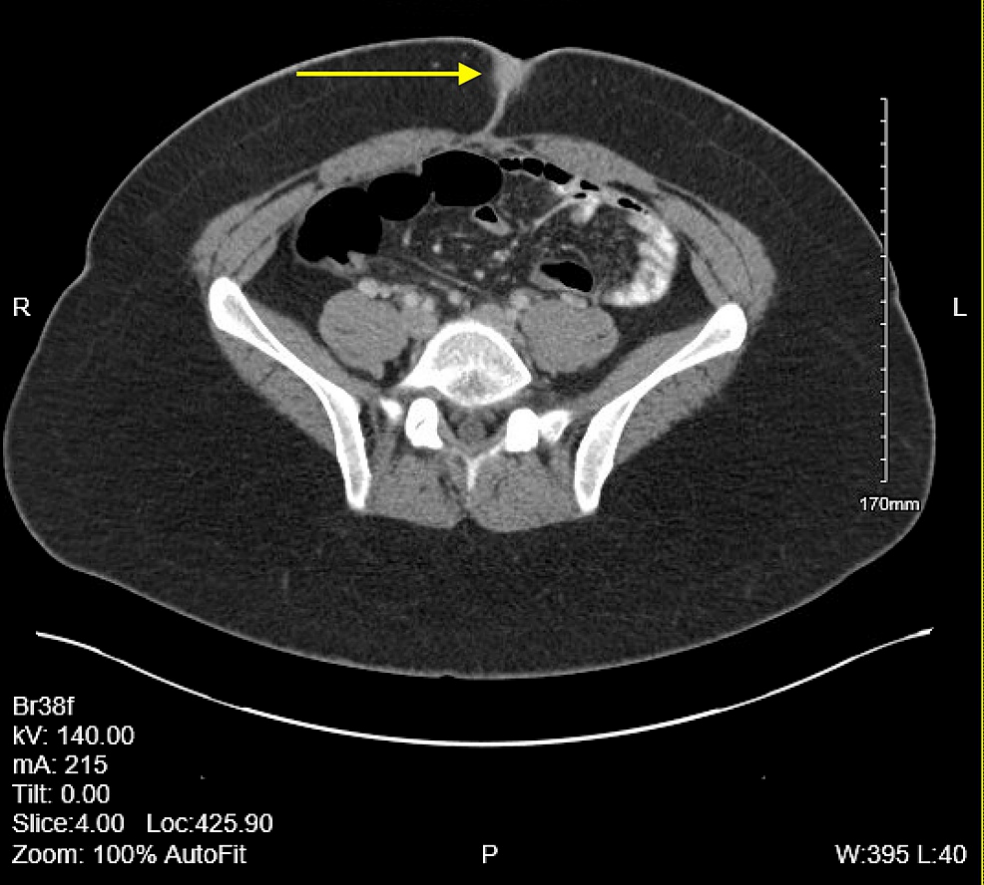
Computerized tomography (CT) scan 4
